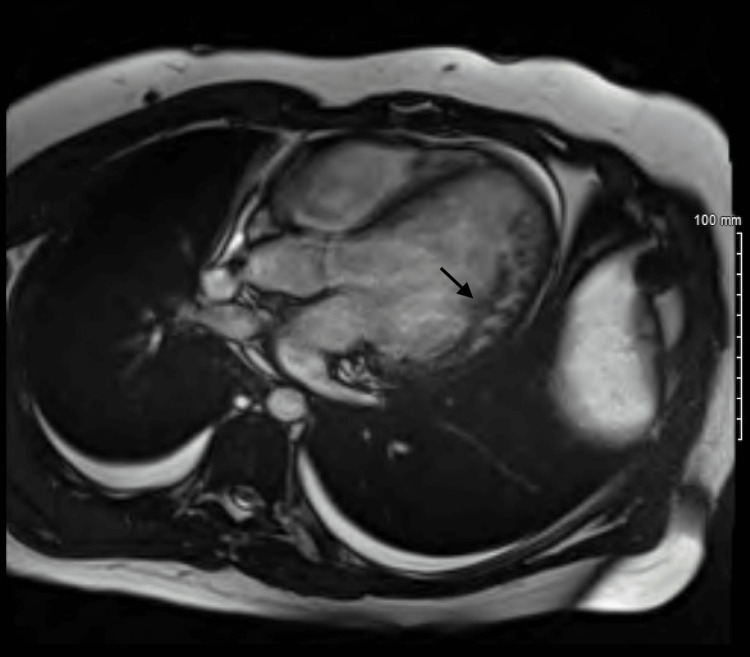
Cardiac magnetic resonance imaging (MRI) showing non-compaction of the left ventricle.
